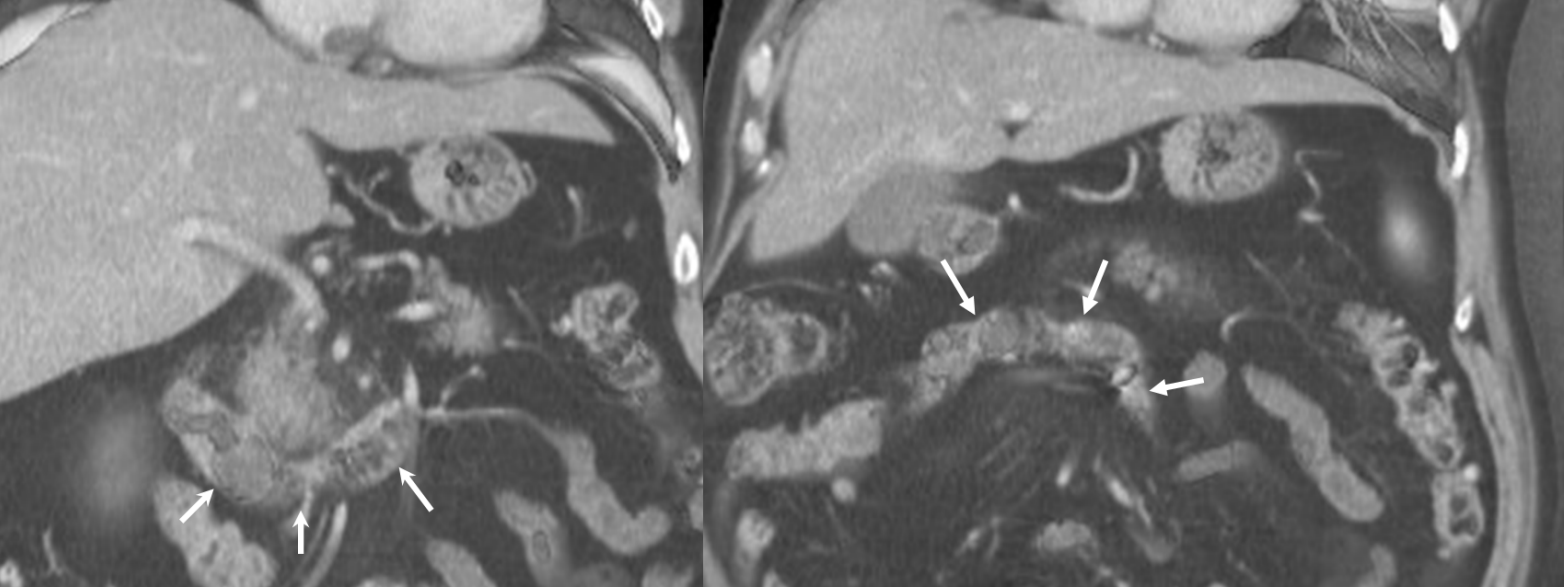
CT, coronal reformatted images demonstrating abnormal rotation of the proximal jejununum, with proximal segment extending horizontally across the midline to the right side of the abdomen (arrows).
